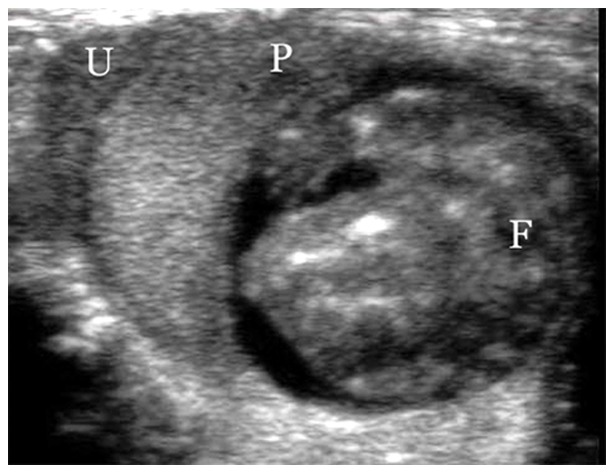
The anatomic structure of uterus (U), placenta (P) and fetus (F) were clearly depicted at 15 day of gestation by conventional ultrasound with 15L8W linear transducer.
